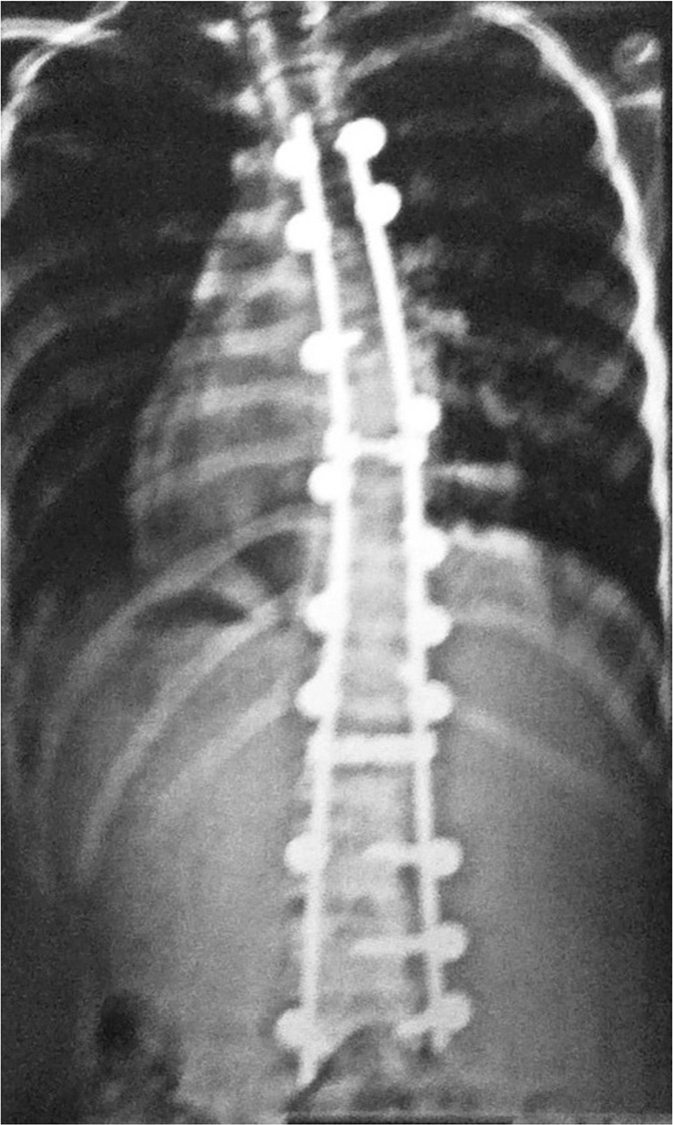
Case 1: The patient after surgery – Radiograph in AP view
